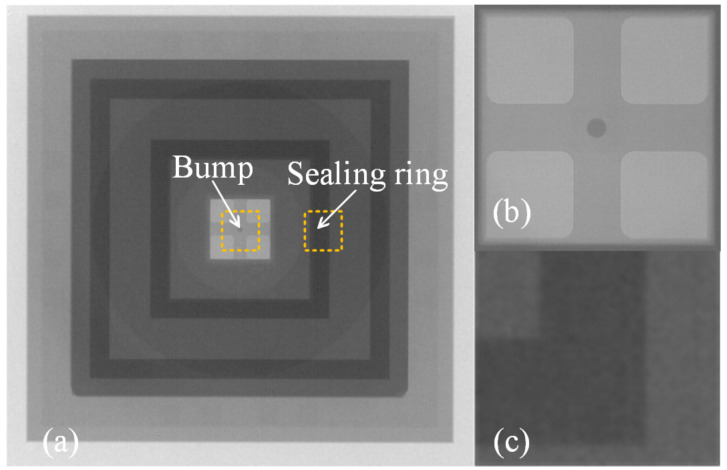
X-ray images. (a) Overall situation. (b) Round bump. (c) Sealing ring.
